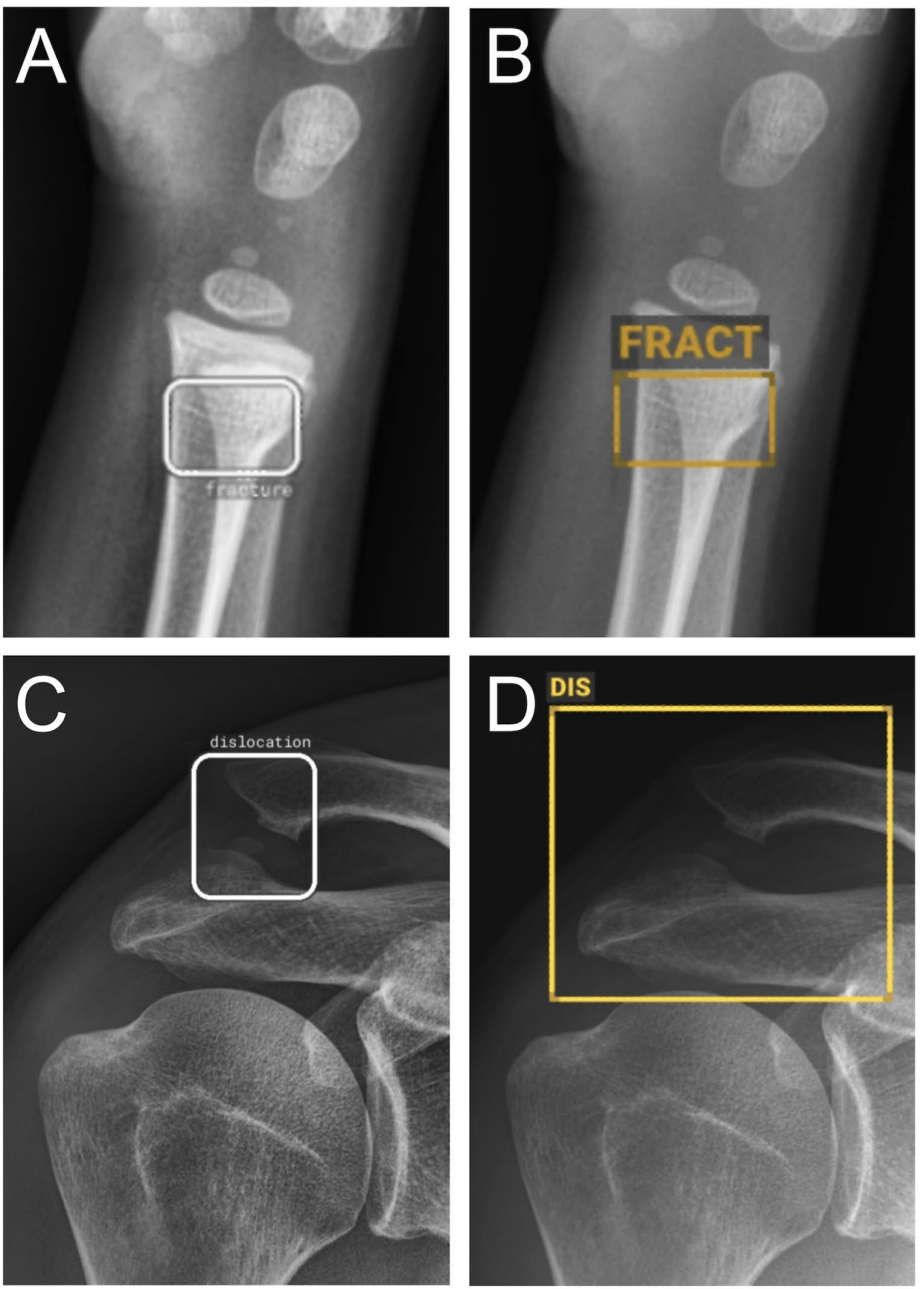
Examples of true positive fracture (top row) and dislocation (bottom row). Lateral radiograph of a 5-year-old patient with distal radius fracture classified as obvious; both RBfracture (A) and BoneView (B) correctly localized the fracture. An adult patient with an AC-joint dislocation was correctly detected by RBfracture (C) and BoneView (D)
